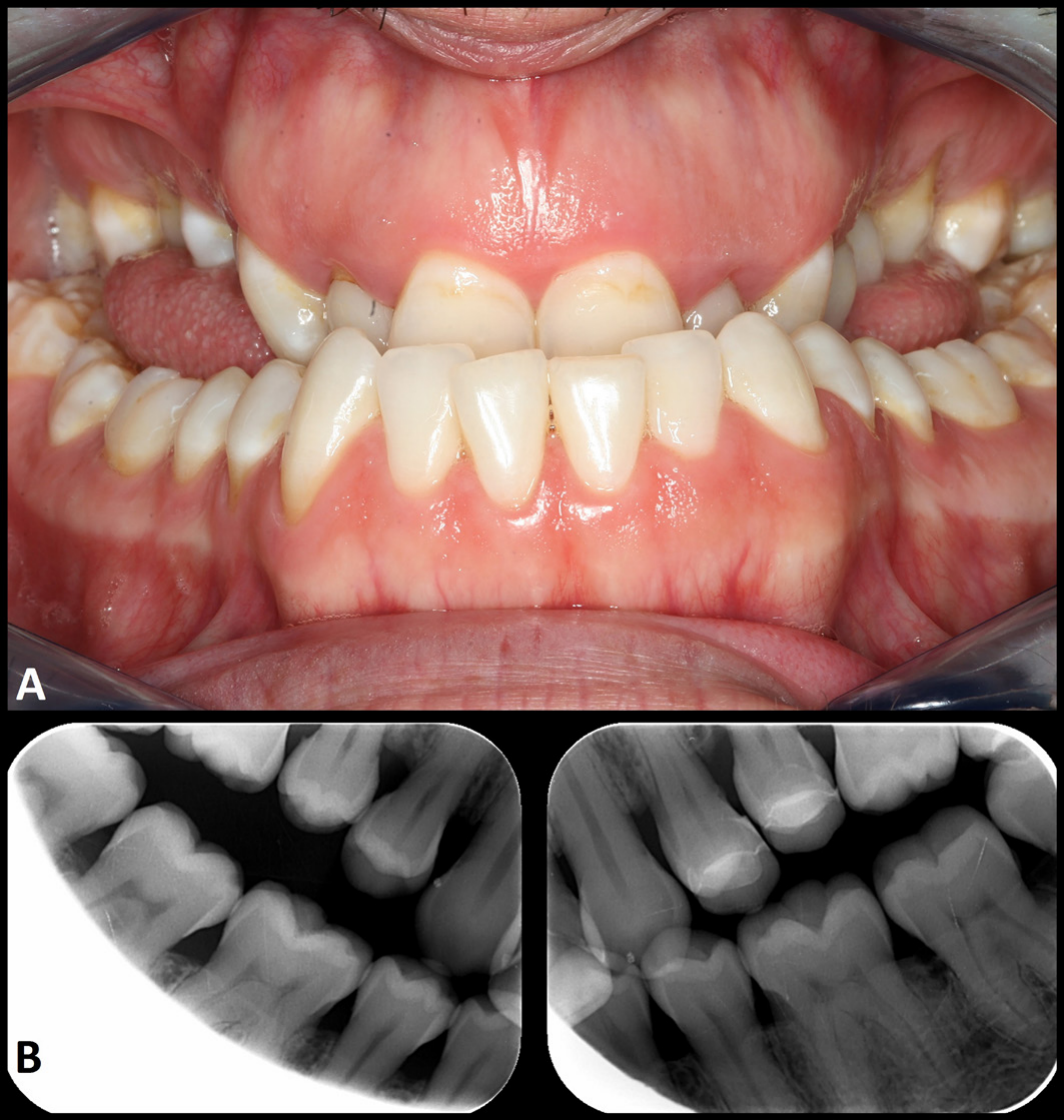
Views of the patient in June 2024, at the age of 36 years. A. Dental occlusion. B. Bite-wing radiographic views.
